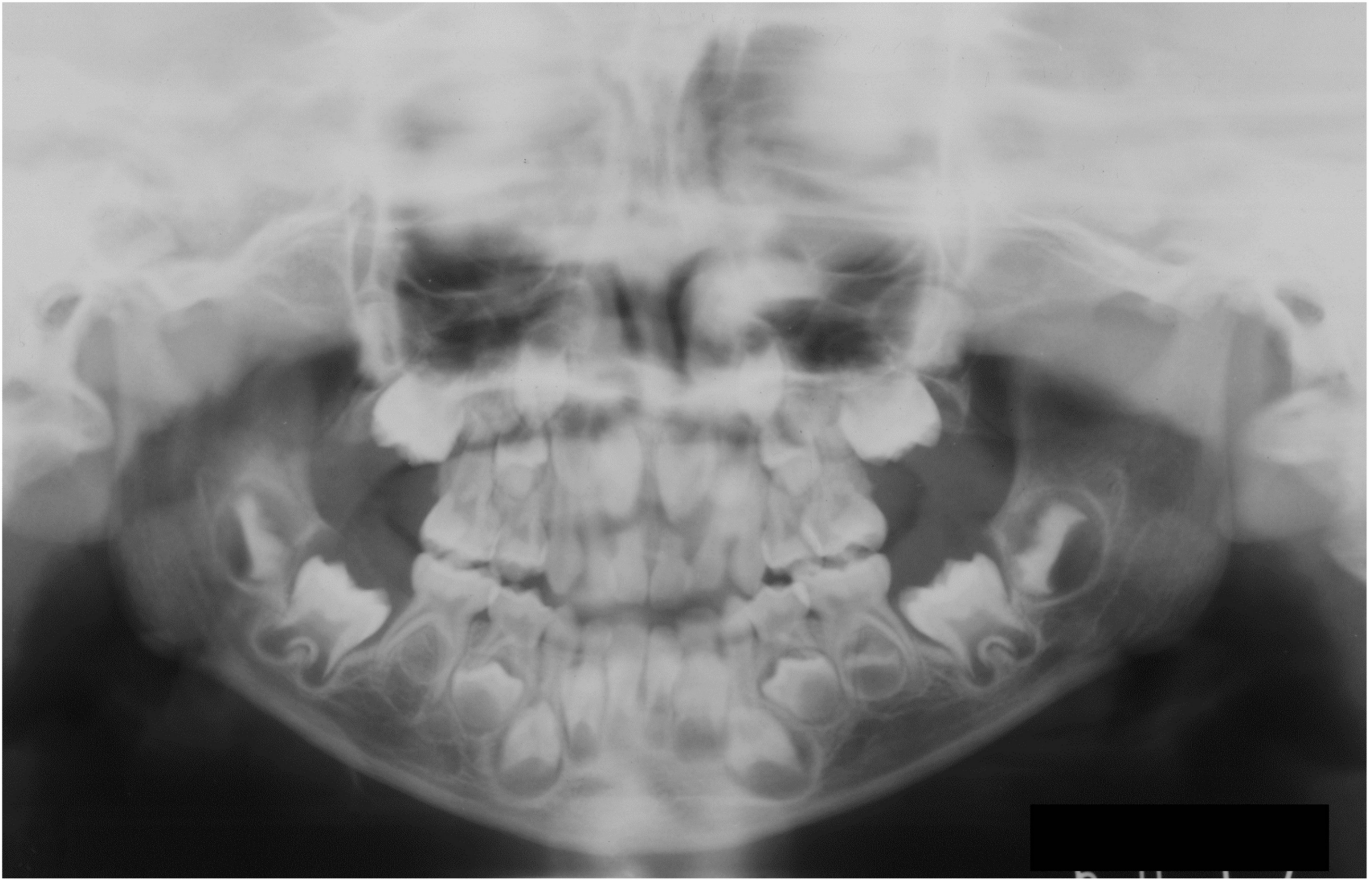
Maxillofacial radiograph at age six No abnormal findings in the maxillofacial bones are observed
